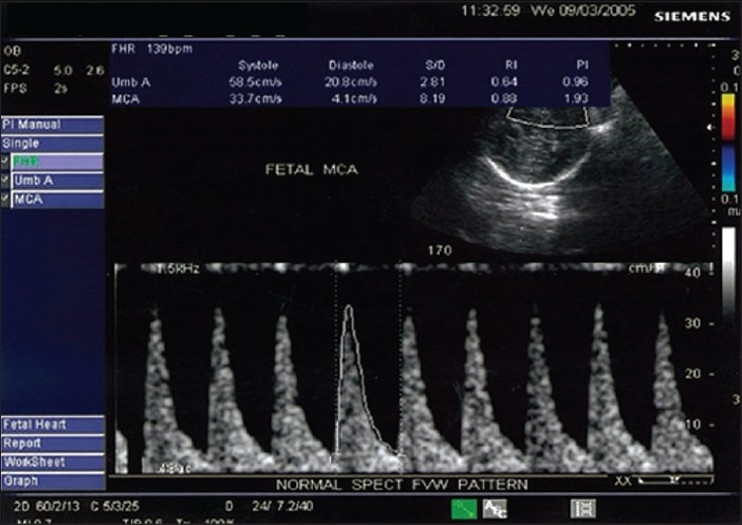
Color Doppler of the normal middle cerebral artery shows a normal MCA waveform pattern, with high resistance, low diastolic flow and increased pulsatility index
